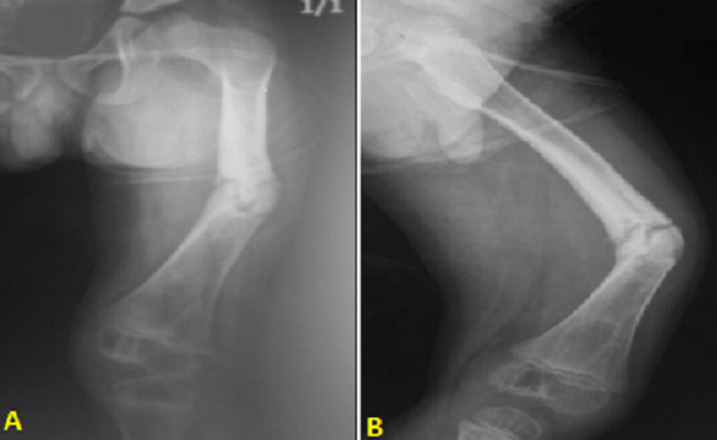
radiographie standard du fémur gauche (A,B): ostéomyélite aiguë de l´extrémité inférieure du fémur compliquée d´une pandiaphysite chronique avec fracture récidivante et raccourcissement par télescopage du foyer fracturaire
